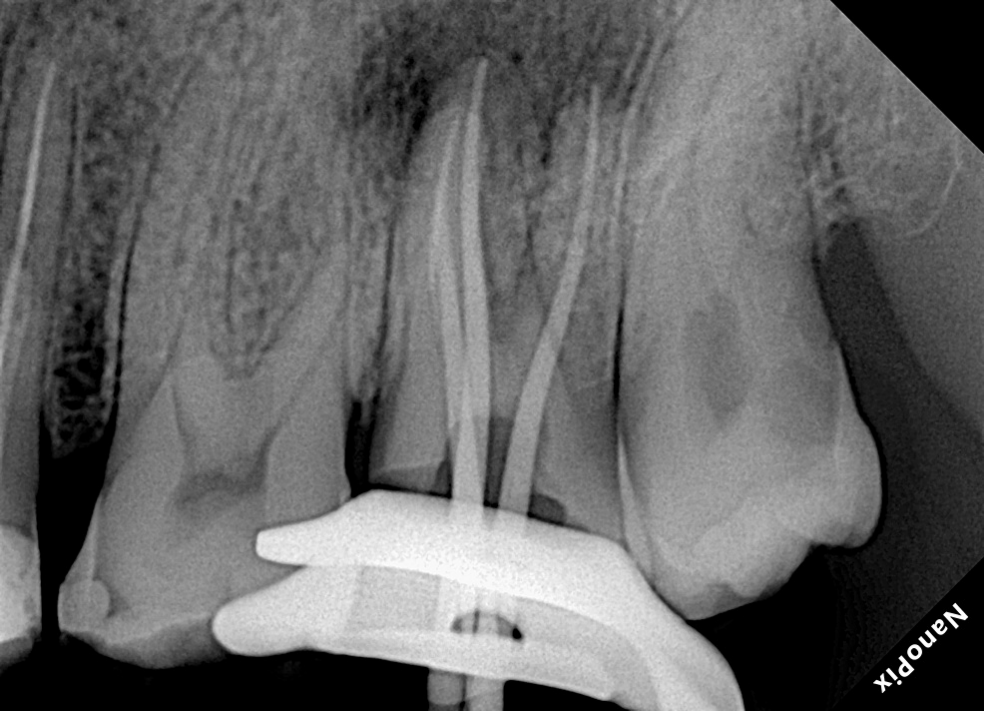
Mid-operative radiograph to show the fit of the gutta-percha cone
